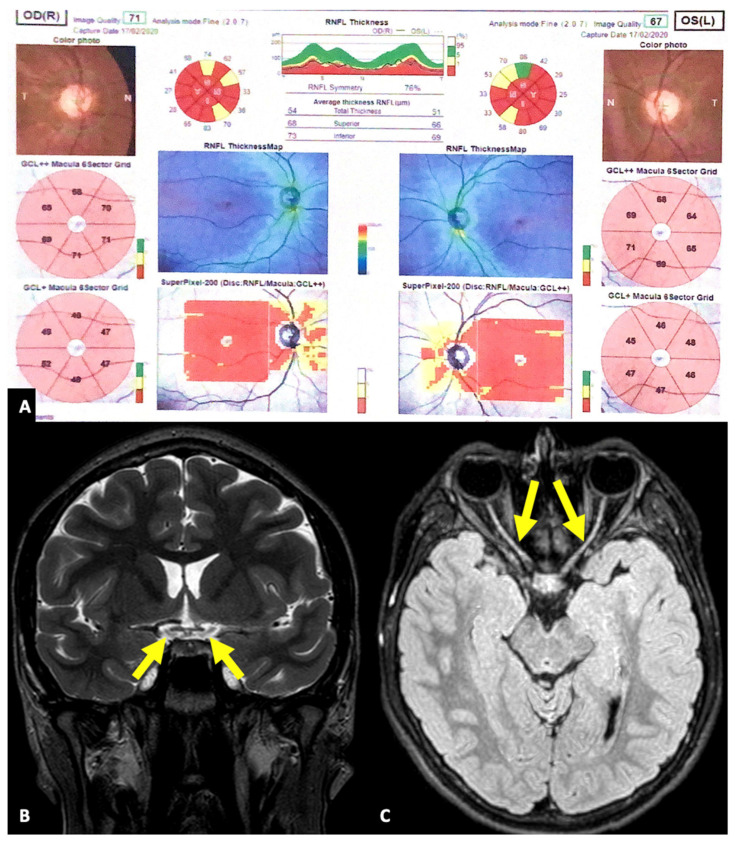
Optic atrophy. Optical coherence tomography (OCT) (A) showed a significant reduction in retinal nerve fiber layer (RNFL) thickness and extensive damage of ganglion cell layer (GCL). Brain magnetic resonance (MR), in the coronal T2 scan (B) and the axial fluid-attenuated inversion recovery (FLAIR) scan (C), showed marked thinning of the optic chiasm (arrows in (B)) and the optic nerves (arrows in (C)). Overall, OCT and brain MR were consistent with bilateral optic atrophy.
